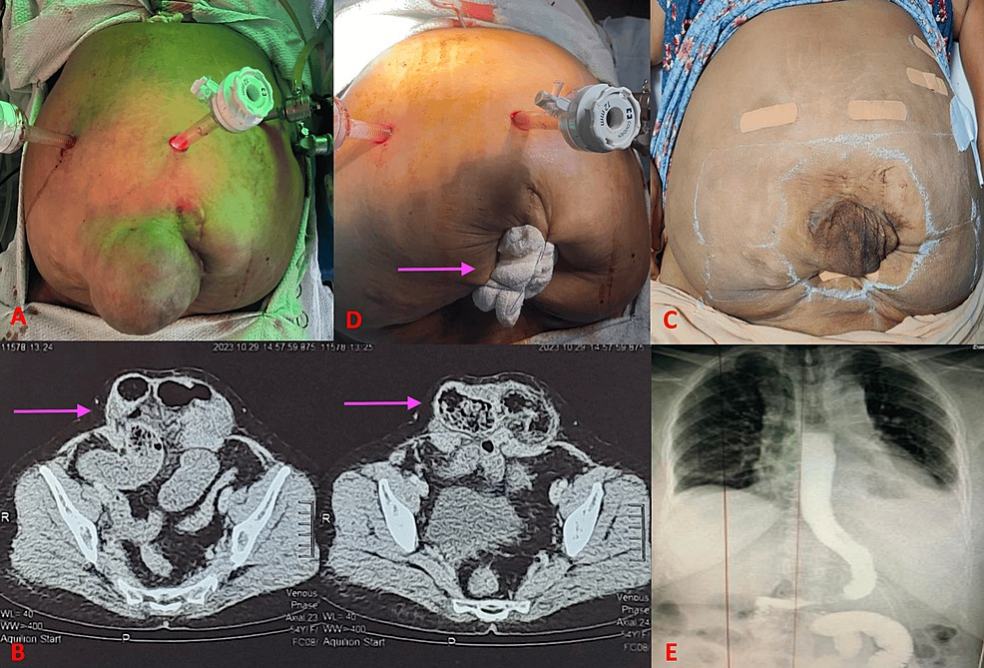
(A) The patient with an infraumbilical incisional hernia with a large sac. (B) CT scan showing the abdominal defect in the lower midline (pink arrows). (C) Port-site positions (taped). (D) Surgical mop plugged and tied with temporary sutures (pink arrow). (E) Gastrograffin X-ray imaging to delineate the post-procedure gastrointestinal anatomy.
